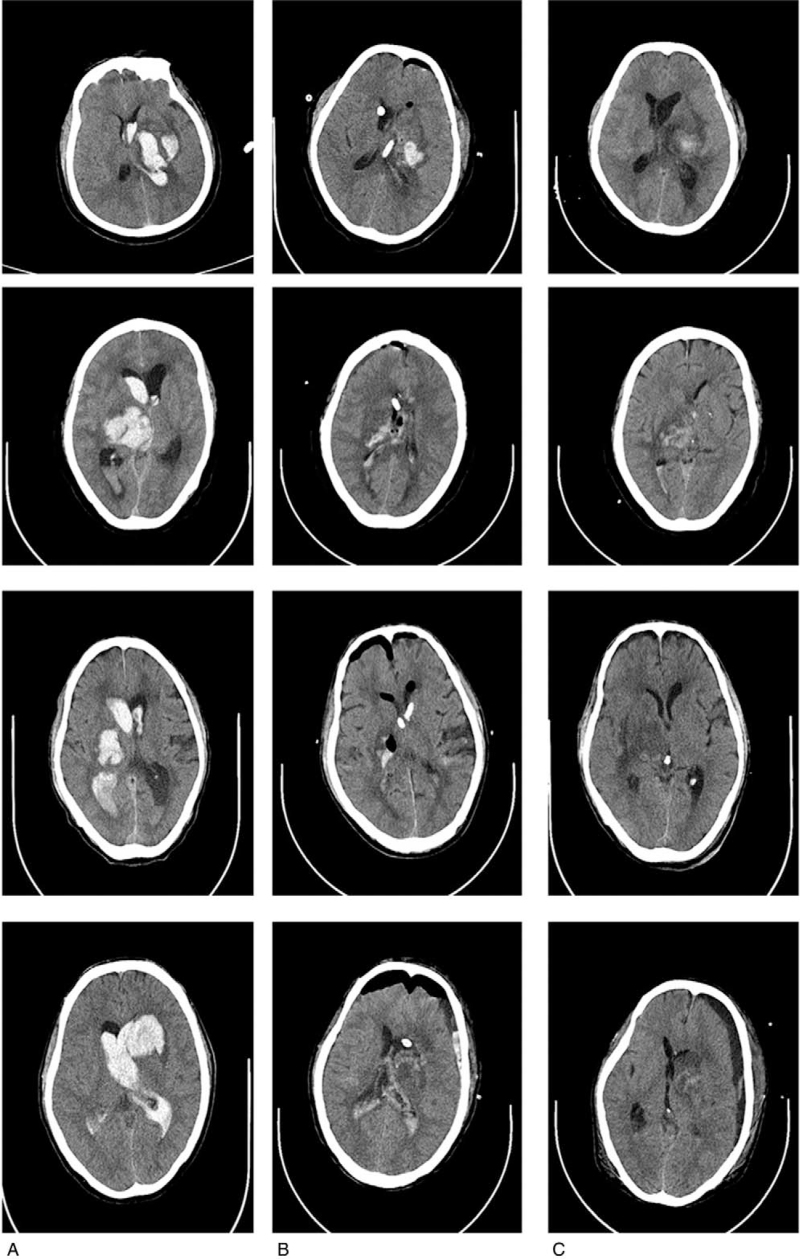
Pre- and postoperative CT scan images of severe IVH treated by transcortical approach surgery. (A) CT scan image at onset, with IVH and ventricular enlargement. (B) CT scan image 12 h after the transcortical approach surgery; the brain hematoma was mostly cleared. (C) CT scan images in the first week after the surgery. The hematoma was absorbed, with no significant edema and other injuries. CT, computed tomography, IVH = intraventricular hemorrhage.
